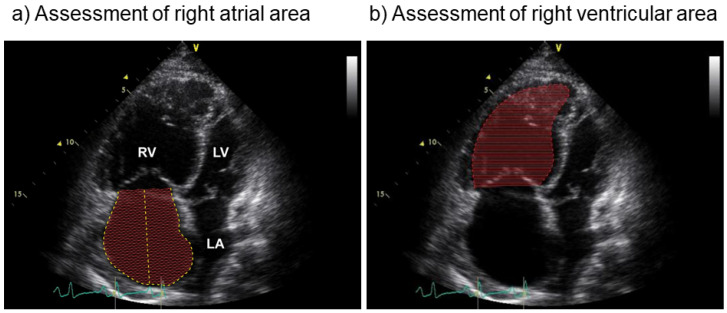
RA (right atrial) and RV (right ventricular) areas are assessed by transthoracic echocardiography in apical 4-chamber view (The 4-chamber view also displays LV (left ventricle) and LA (left atrium). For the assessment, the margins of the right atrium and the right ventricle are traced to calculate their area. In this figure, RA (a) and RV (b) areas are shown filled out with lines.
